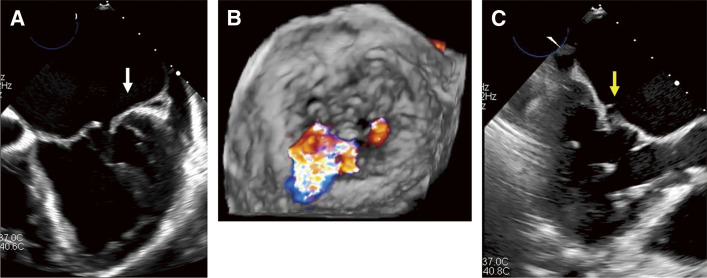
Preoperative transesophageal echocardiographic images. (A) Movement of the posterior mitral leaflet was restricted (white arrow). (B) A surgeon’s view. The mitral regurgitant jet came from the anterolateral P2 area. (C) An abnormal tissue appeared and disappeared on the posterior mitral leaflet along with the heart beating (yellow arrow).
